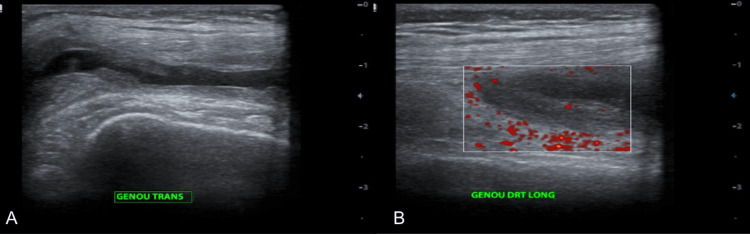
Musculoskeletal ultrasound of the right knee Demonstrating grade III chronic synovitis (A) with a negative Doppler signal (B), synovial hypertrophy with synovial fringes.
